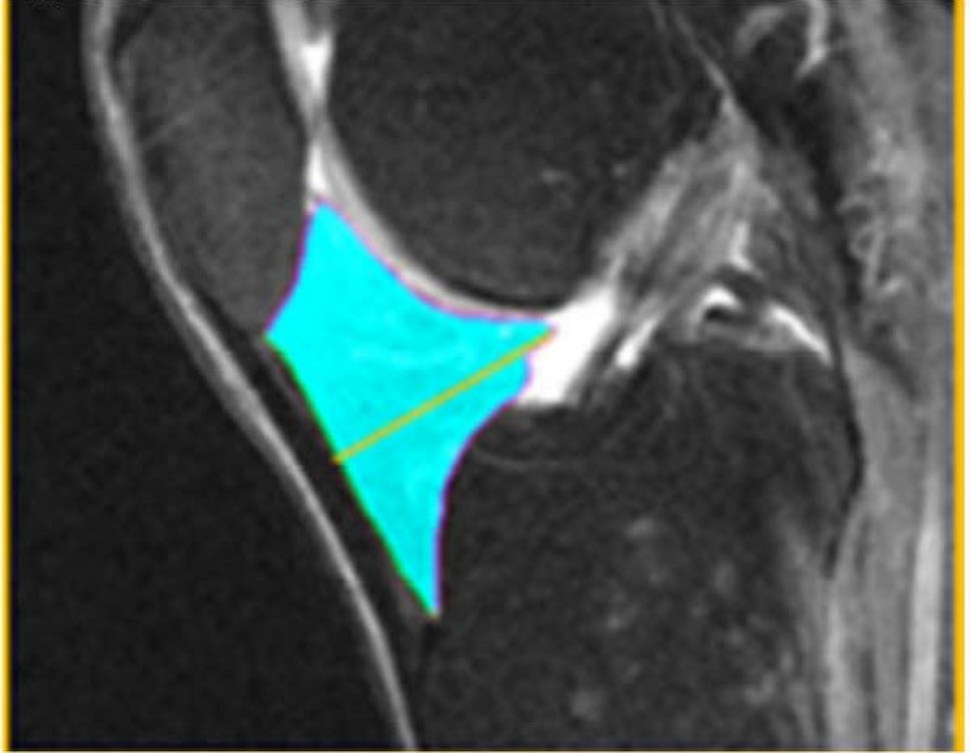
Fat pad maximal sagittal thickness. Manual measurement on 3T sagittal IW 2D TSE Fat-suppressed MRI sequence
